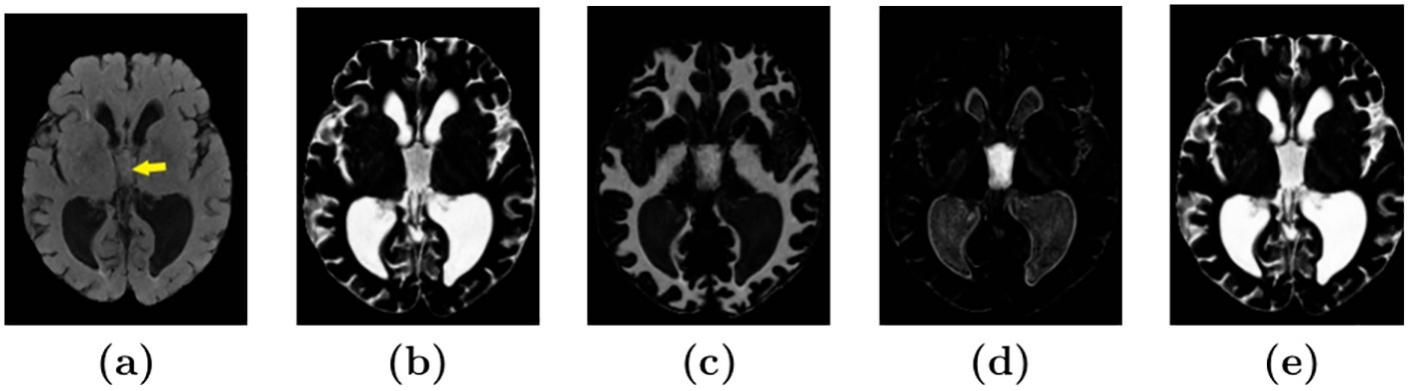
Identification and removal of pulsation artifact. Image (a) shows a FLAIR image with a pulsation artifact in the third ventricle (yellow arrow). Images (b) and (c) show the corresponding CSF and WMH output, respectively, from SegAE before thresholding. Image (d) shows a pulsation artifact segmentation obtained with element-wise multiplication of the CSF and WMH outputs (non-binarized). Image (e) shows the CSF segmentation that has been corrected for pulsation artifacts by adding the pulsation artifact segmentation shown in (d).
